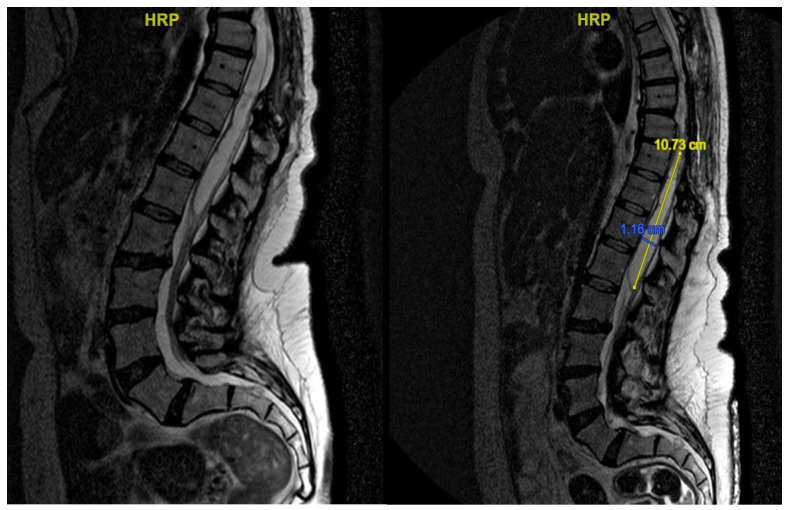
Case 4: post-operative MRI showing the reduction in syringomielic size: pre-operative (left) and post-operative (right).
